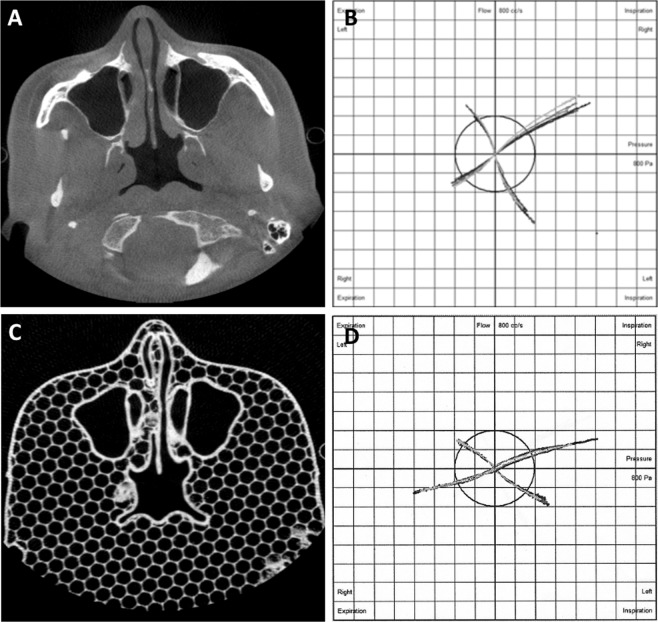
Corresponding results *in vivo* and *in vitro*. Above: Axial CBCT image (A) from a patient and rhinomanometry (B) from the same patient. Below: Corresponding CBCT image (C) and rhinomanometry (D) from the 3D print.
